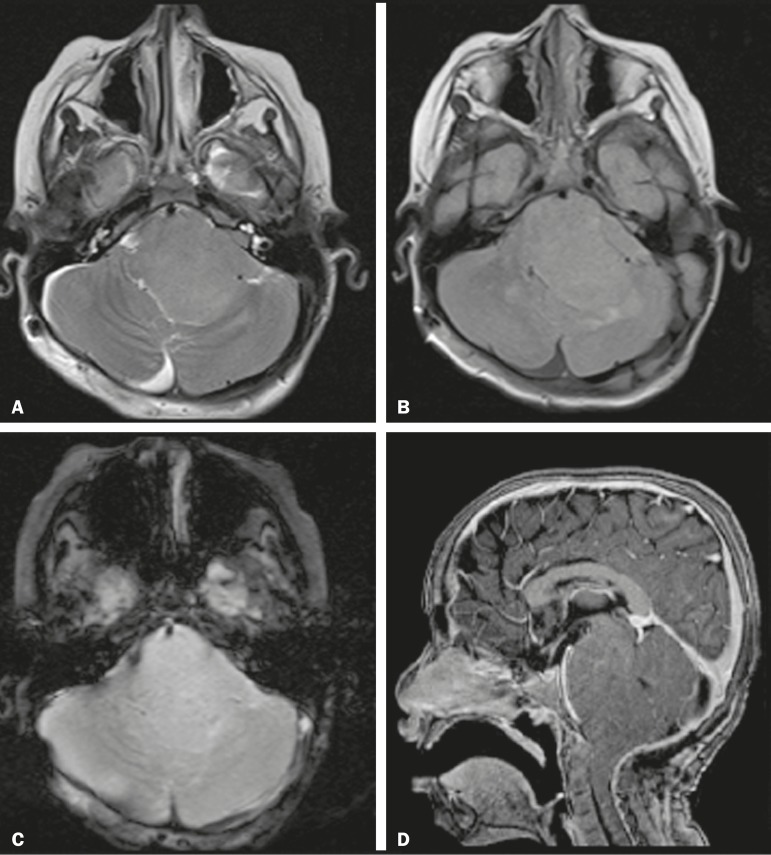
Magnetic resonance imaging. Axial T2-weighted sequence (A), axial T2-FLAIR sequence (B), axial T2*-weighted sequence (C), and contrast-enhanced sagittal T1-weighted sequence (D), showing an expansile, infiltrative lesion centered at the pontobulbar junction, with a slightly hyperintense signal in the T2-weighted sequences, although without cysts, calcifications, or contrast enhancement.
